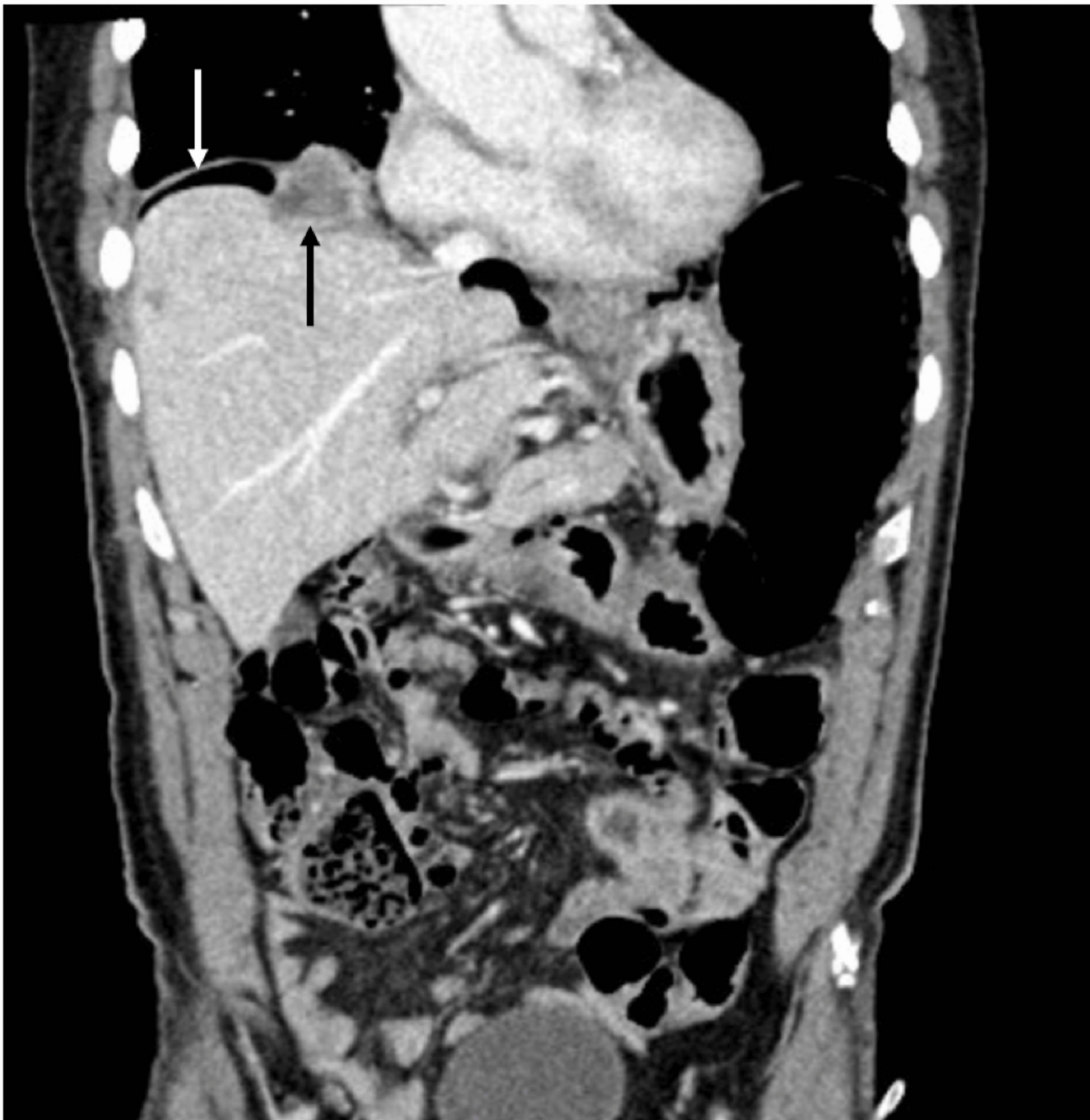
Coronal view of CT abdomen pelvis showing right-sided diaphragmatic lesion (black arrow) with pneumoperitoneum (white arrow)
